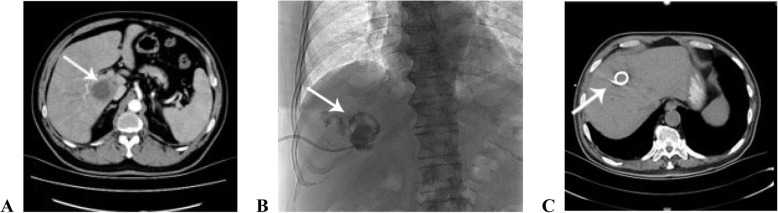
Clinical features of liver abscess. a Contrast-enhanced abdominal CT image of liver abscess suggestive of intrahepatic abscess. b Plain abdominal radiograph of the liver abscess after drainage, showing that the drainage catheter was well-positioned, and the abscess had subsided. c Contrast-enhanced abdominal CT image of the liver abscess after drainage, showing that the drainage catheter was well-positioned, and the abscess had subsided
